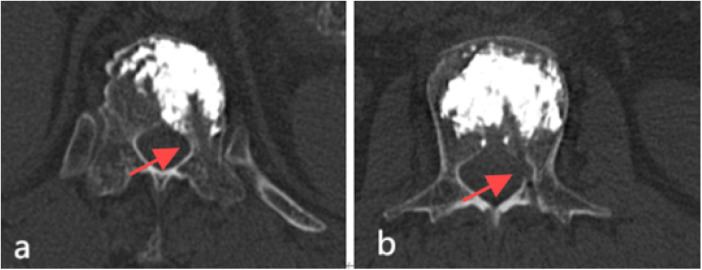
Evaluation of puncture trajectory accuracy via postoperative axial CT. (a) Puncture trajectory completely contained within the pedicle cortex; (b) puncture trajectory breached the medial pedicle wall (red arrow).
